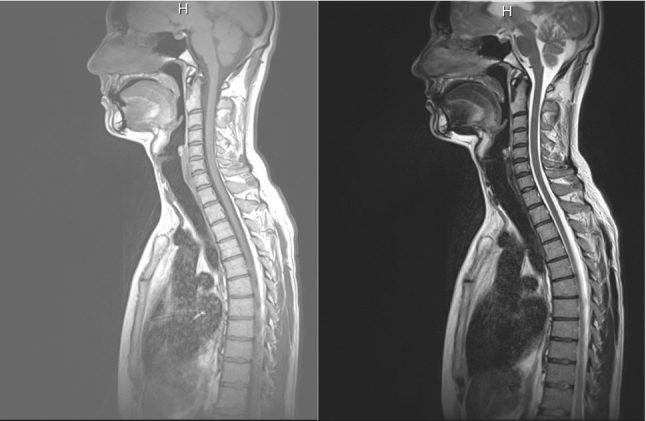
Example slices from T1-weighted (left) and T2 (right) sequences
